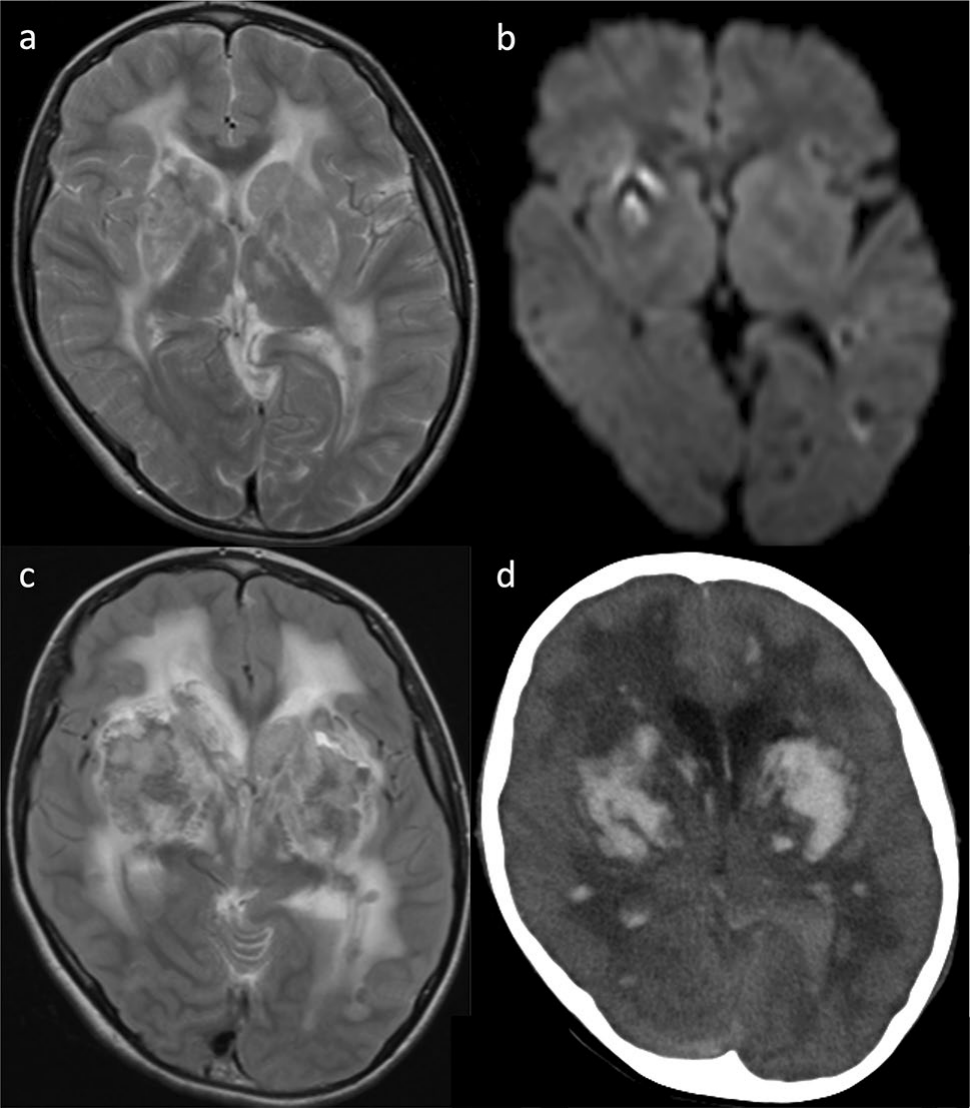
COLGALT1*-related disorder. Axial T2-weighted imaging (a) at the age of 9 years demonstrated hyperintense lesions in the bilateral basal ganglia, thalami, deep cerebral white matter, and internal and external capsules. Axial diffusion-weighted imaging (DWI) (b) revealed susceptibility effects of microbleeds in the right basal ganglia and left temporal white matter. After 1 day, axial FLAIR imaging (c) and computed tomography (d) after the patient lost consciousness owing to an influenza virus infection demonstrated acute, massive, bilateral, parenchymal and intraventricular hemorrhages (reprinted with permission from Reference [35])
